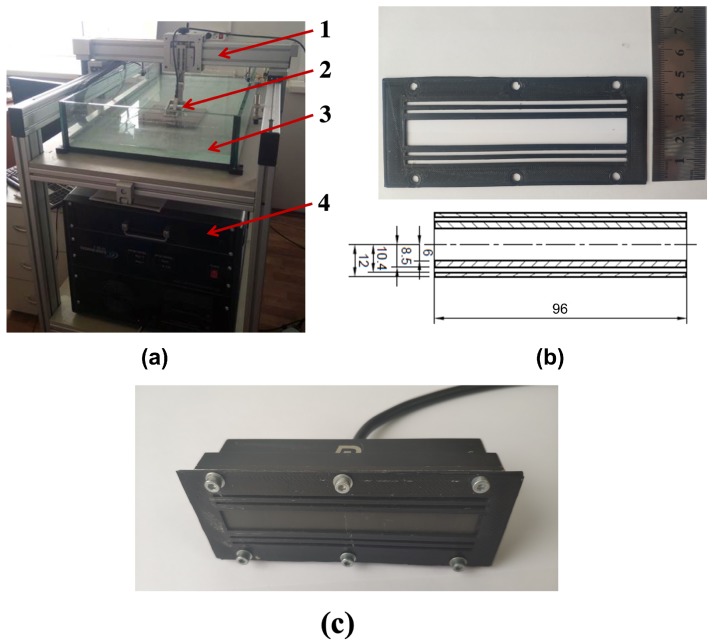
Photo of (a) Idealsystem3D experimental set-up, where 1 is the ultrasonic scanner, 2 PA, 3 immersion bath and 4 electronic unit. Photo and scheme of (b) manufactured PR-FZP. Photo of the lens (c) mounted in the PA.
